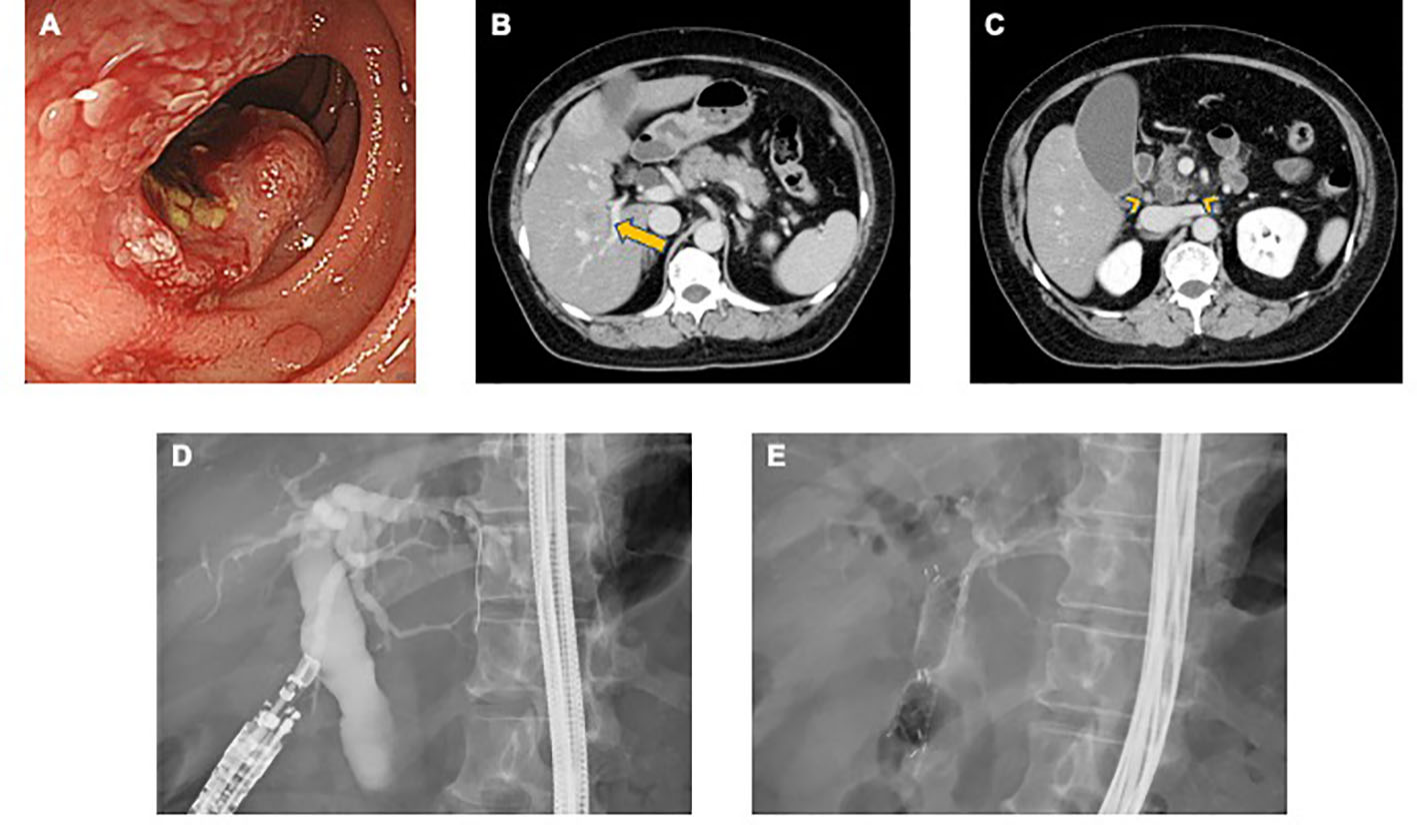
(A) Endoscopic findings of the duodenum reveal a type 2 tumor on the descending part. (B, C) Abdominal computed tomography shows multiple liver metastases (long arrow) and lymph nodes (arrowhead). (D) After EUS-guided puncture, contrast agent was injected into the common bile duct for cholangiogram. (E) Choledochoduodenostomy was accomplished with a fully covered metallic stent through the duodenal bulb.
